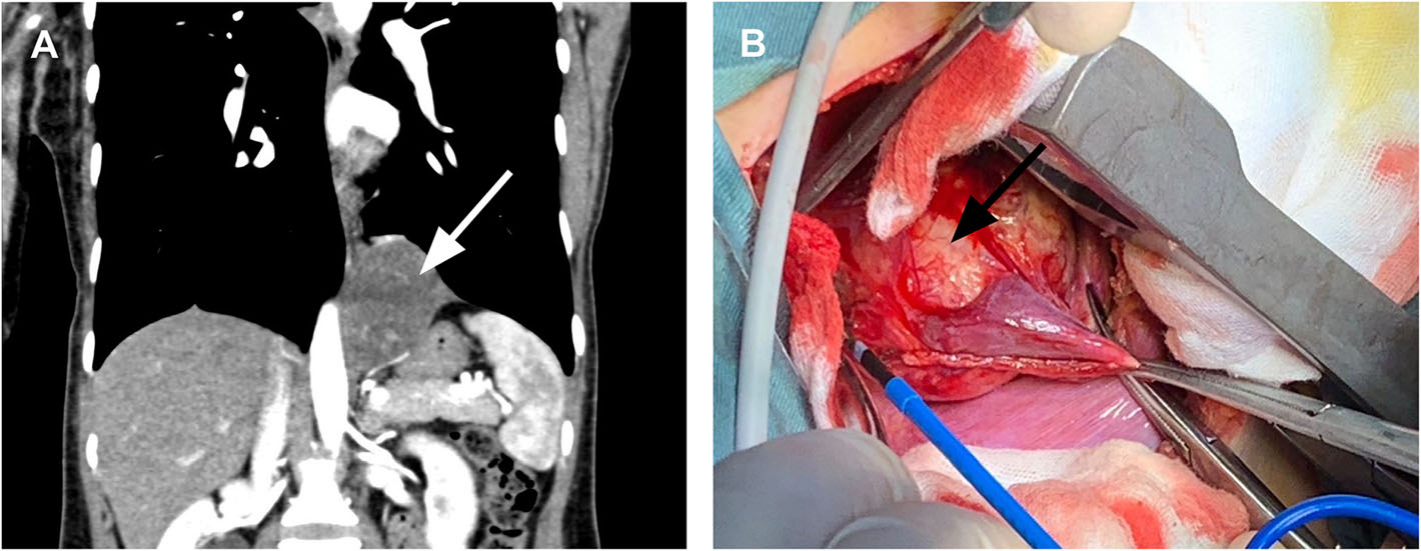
a CT images showing the tumour (white arrow) was enhanced and was found to be closely related to the oesophagus and diaphragm. b Intraoperative photographs showing the tumour (black arrow) originating from the diaphragm and adhering to the left lower lung
